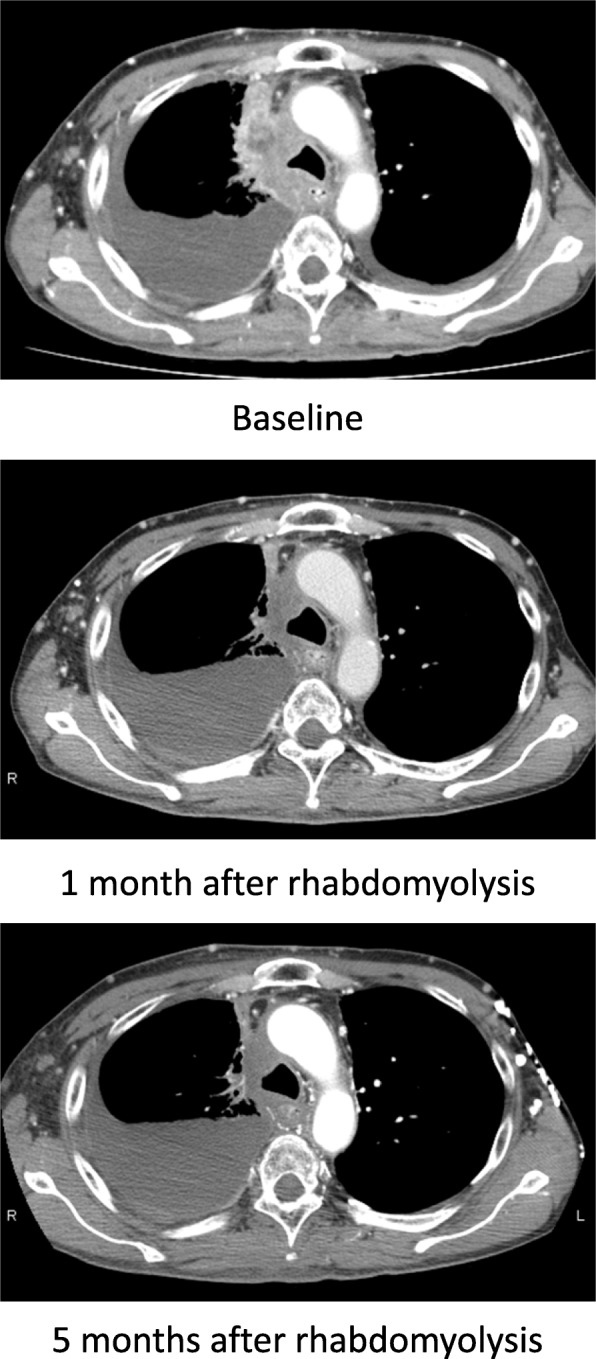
Computed tomography images of NSCLC. These images show maintained tumor shrinkage after reducing the doses of dabrafenib and trametinib
